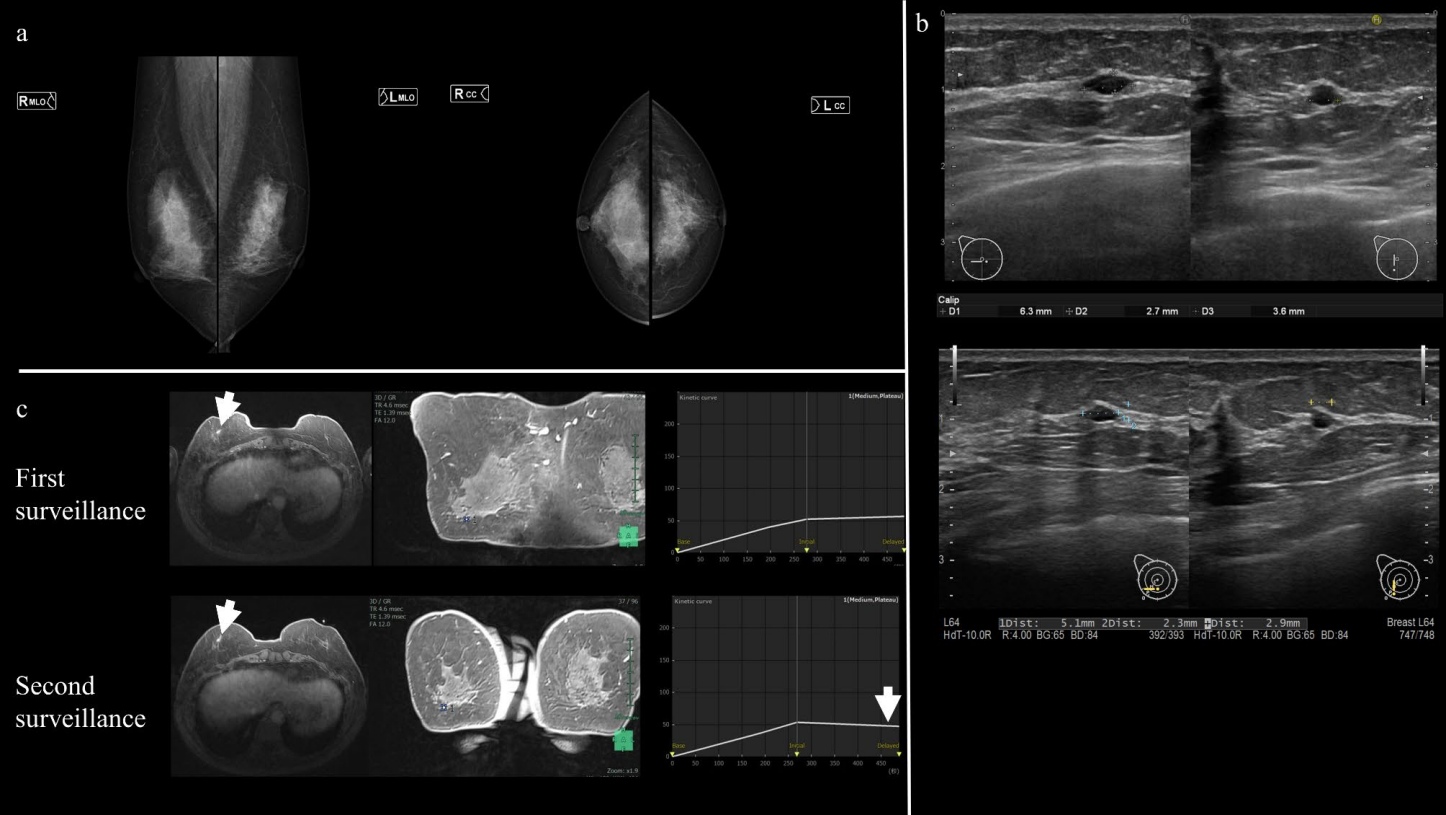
Breast imaging. (a) Mammography shows no malignant findings. Bilateral diffuse microcircular calcifications are observed in both breasts, but no significant differences from the previous imaging are apparent. The case is classified as category 2. (b) Breast echography shows a well-defined nodule in the lower outer quadrant on right breast surveillance before starting breast surveillance (top; approximately 2 years and 10 months previously) and before performing core needle biopsy (bottom). After 2 years and 10 months, almost no change is evident. (c) Contrast-enhanced MRI of the breast taken during the first surveillance (top) and the second surveillance (bottom). Both MRIs show similar nodules in the right breast, but washout is present during the second surveillance that is not seen on the kinetic curve from the first surveillance (white arrow)
